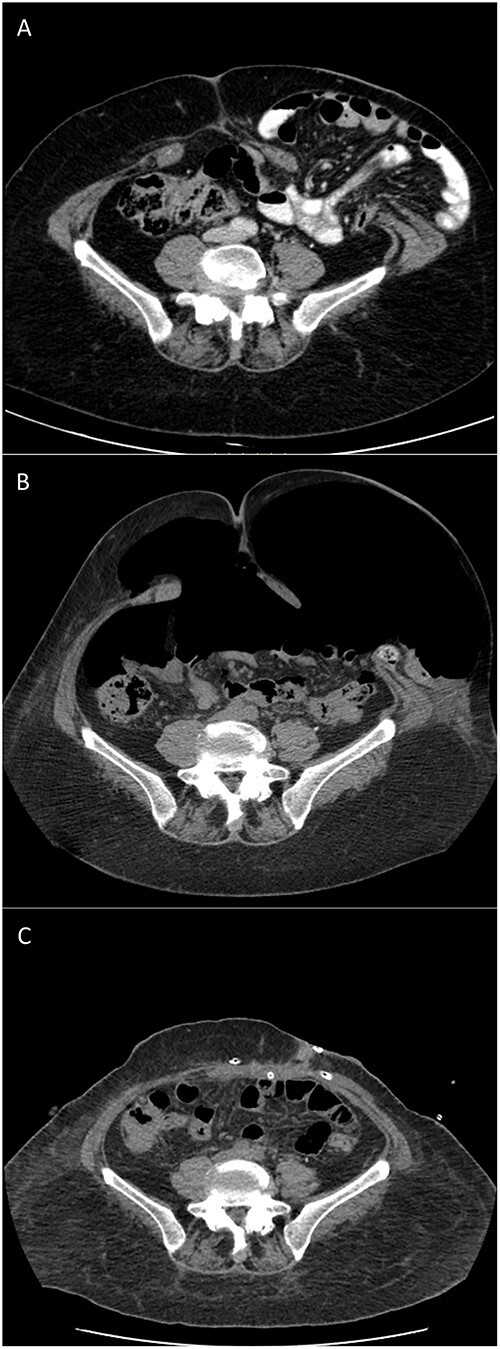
CT scans of type IV PH repair after botulinum toxin and PPP: (A) 9 months before surgery; (B) after preoperative BTA and PPP completion; and (C) 3 days postoperative.
